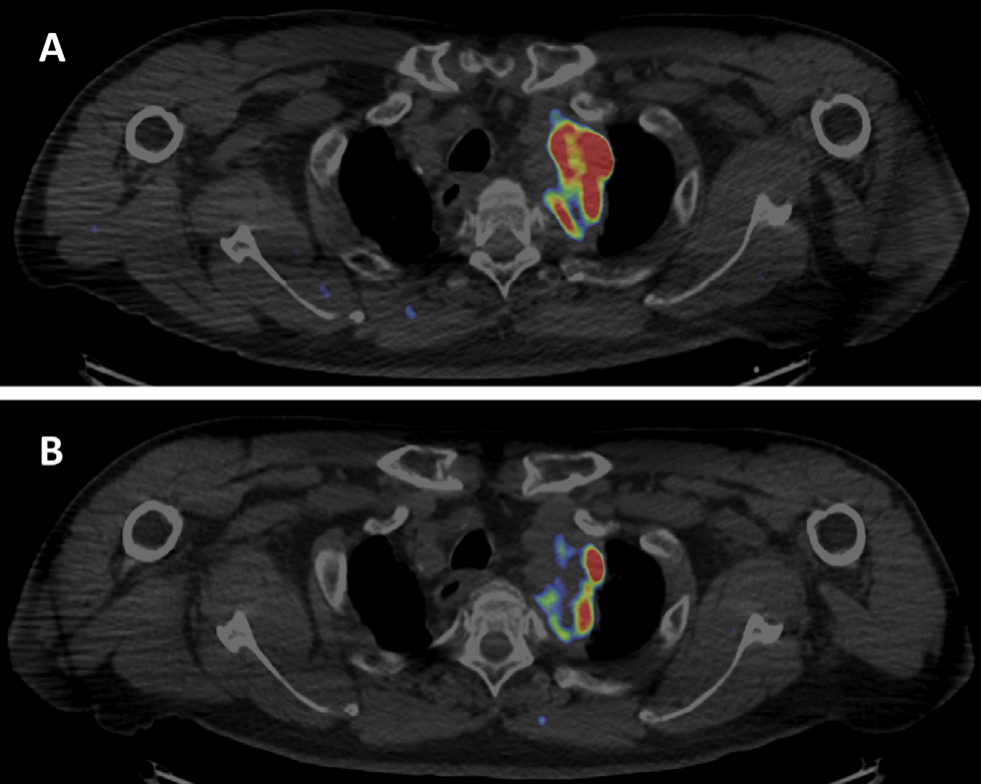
Example of tumour hypoxic response. FMISO PET-CT images for one patient in the expansion cohort before (A) and after (B) buparlisib treatment. PET images are fused with the corresponding CT and displayed on a tumour-to-blood uptake ratio (TBR) colour scale. Red regions depict a TBR greater than 1.4, indicating hypoxia, and no visible PET tracer uptake depicts a TBR below 1, indicating normoxia. In this case, there was a 42% reduction in tumour hypoxic volume after buparlisib. FMISO, ^18^F-fluoromisonidazole; PET, positron-emission tomography; CT, computed tomography.
